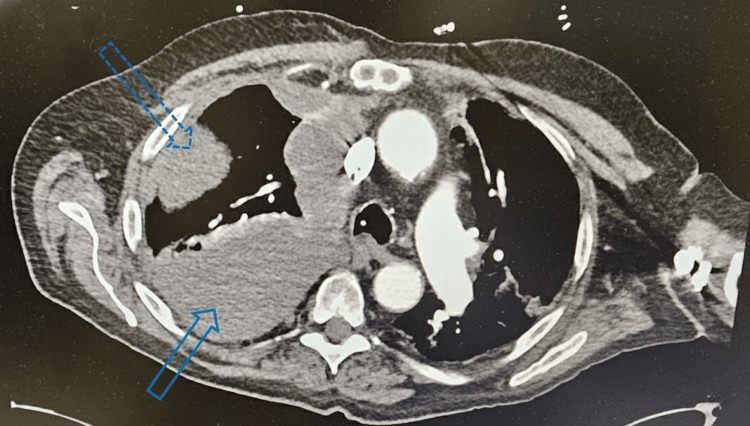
CT scan showing increased right pleural effusion with peripheral pleural changes The solid blue arrow at the bottom of the screen points towards increased right pleural effusion posteriorly versus previous CT scans. The dotted blue arrow at the top of the screen shows an increasingly significant peripheral change versus previous CT scans CT: computed tomography
